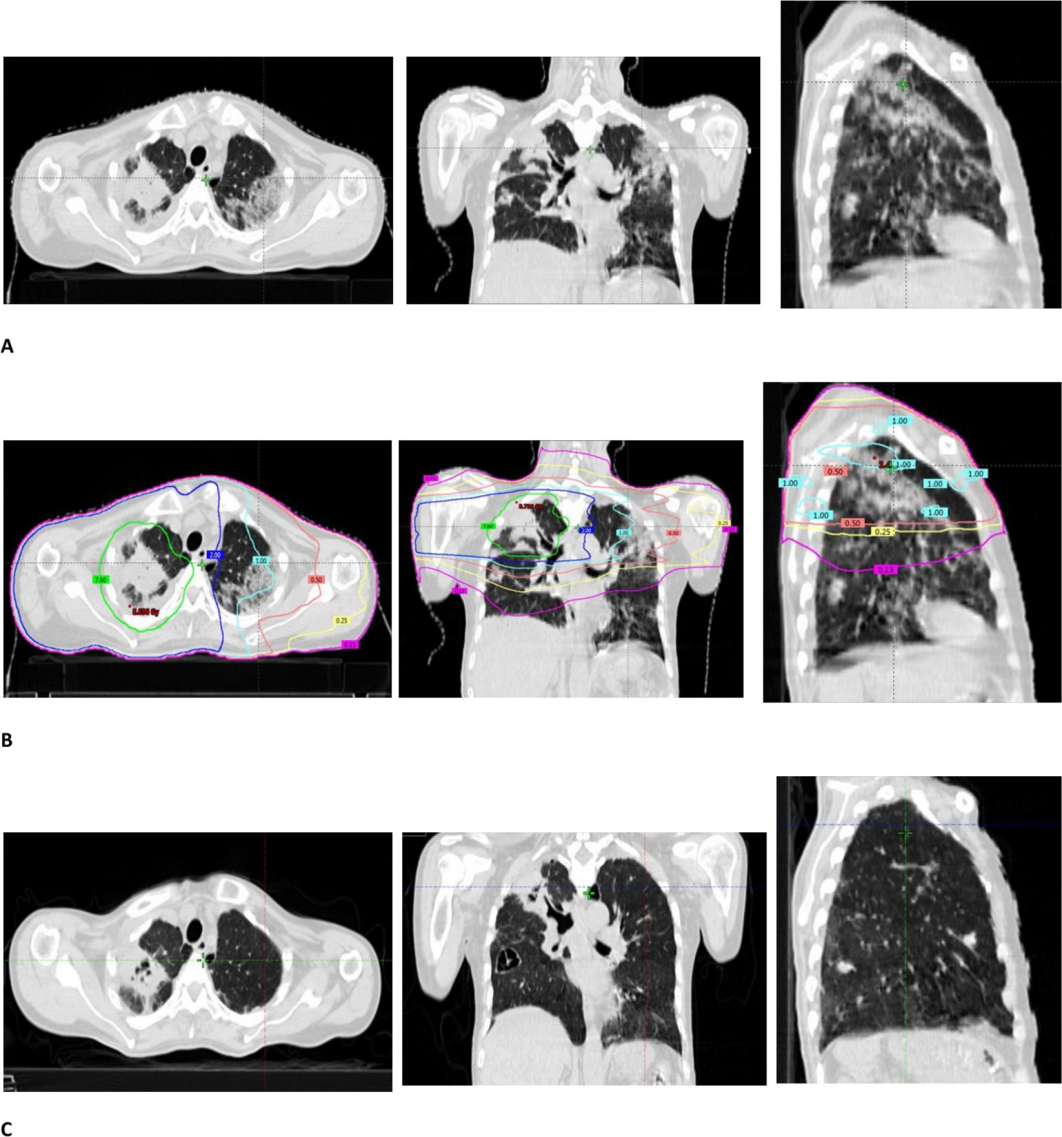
A HRCT scan chest done on 21 November 2020 showed right sided primary lung tumour and bilateral COVID-19 lung lesions (CT-SS 14/25); (B) It shows radiation dose distribution around primary lung lesion (Gross tumour volume +5 mm PTV) and low-dose radiation spillage in bilateral lungs. Right-sided upper lobe lesion received palliative radiation – 8 Gy/1 fraction with volumetric modulated arc therapy (VMAT). [Green colour represents (D_95_) (7.6 Gy) of 8 Gy delivered to primary lung lesion; Low-dose radiation spillage is represented by dark blue 2 Gy isodose; cyan 1 Gy and pink 0.50 Gy, yellow 0.25 Gy and magenta 0.13 Gy in different areas of both the lungs]; (C,) 1-week post-radiation therapy response in bilateral COVID lung lesions attributed to low-dose radiation spillage and CT-SS score declining to 3/25.
